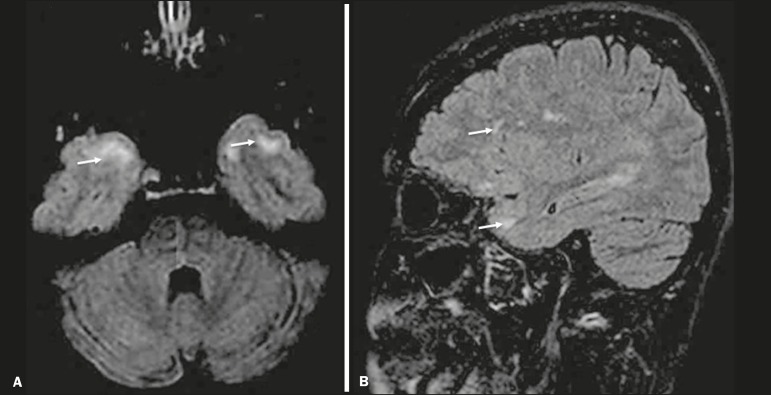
CADASIL. Axial and sagittal FLAIR sequences (A and B, respectively), showing areas of hyperintense signal in the subcortical and anterior portions of the temporal lobes.
